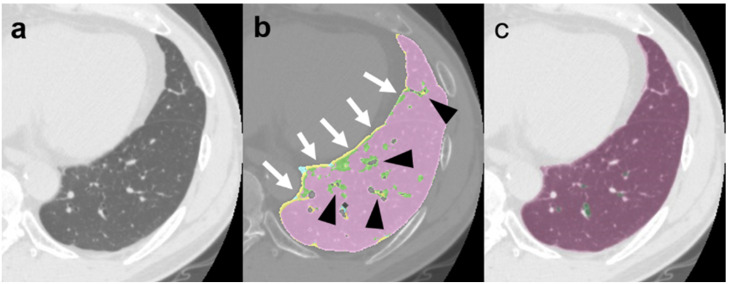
Images of a 57-year-old man with normal lungs. (a) Computed tomography (CT) image; (b) computer-aided diagnosis (CAD) method image; (c) deep-learning (DL)-based image. The conventional CT image (a) shows a normal lung. On the CAD method image (b), the pulmonary surface (white arrow) and perivascular area (black arrowhead) were misclassified as ground-glass opacity (G_CAD_) and honeycombing (H_CAD_), respectively. The DL-based analysis image (c) accurately classifies the normal lung area. The color code follows that described in the legend of Figure 2.
